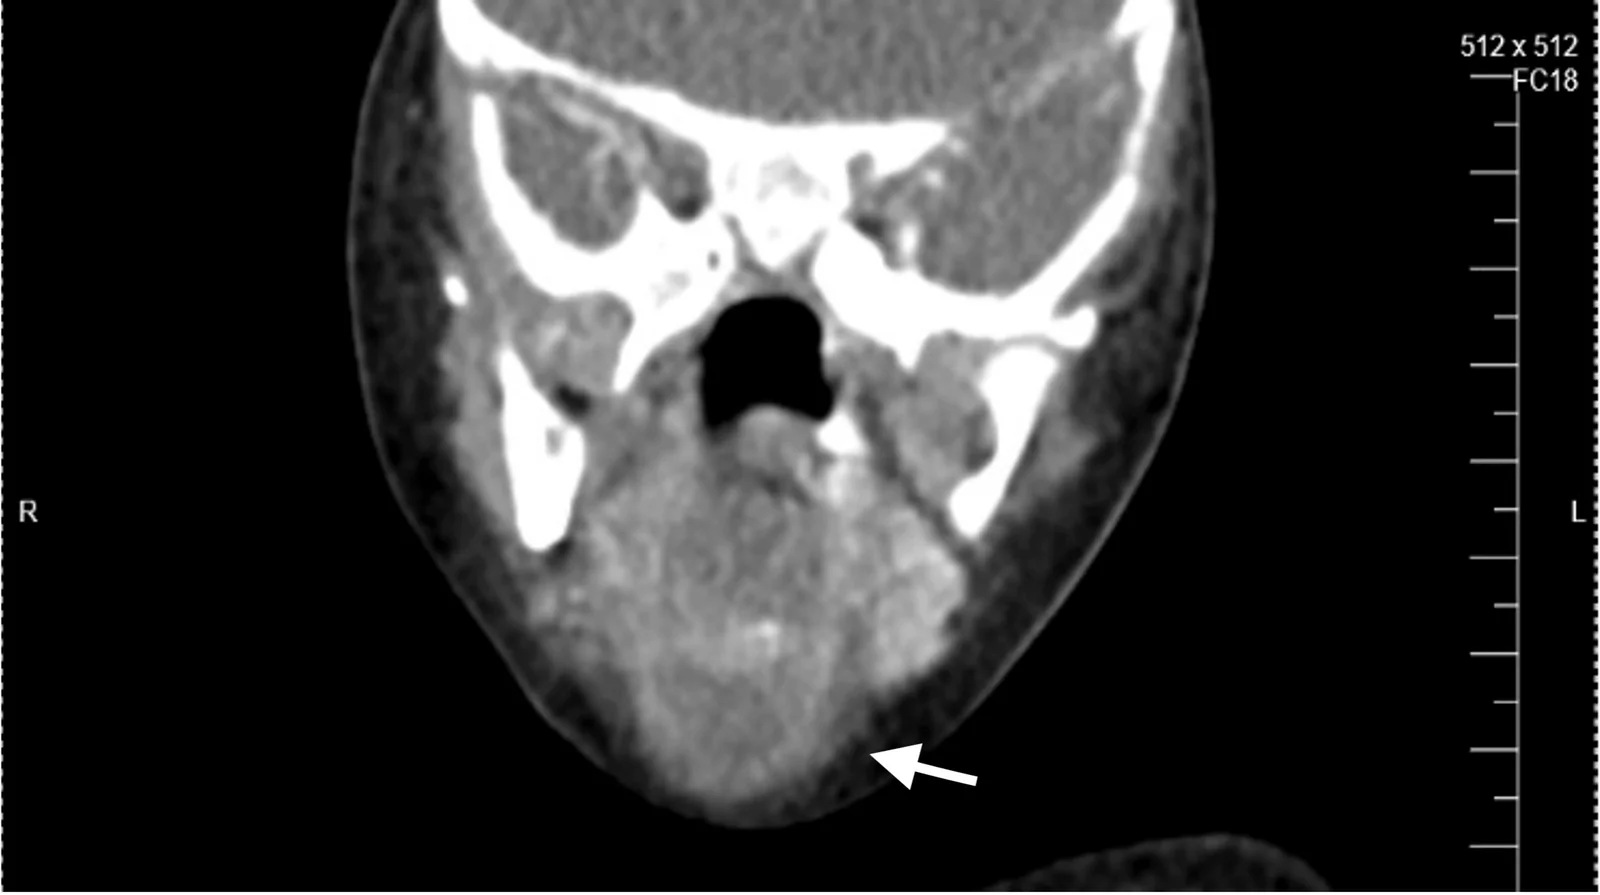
Coronal computed tomography (CT) image of the vallecular cyst (arrow) before drainage.
